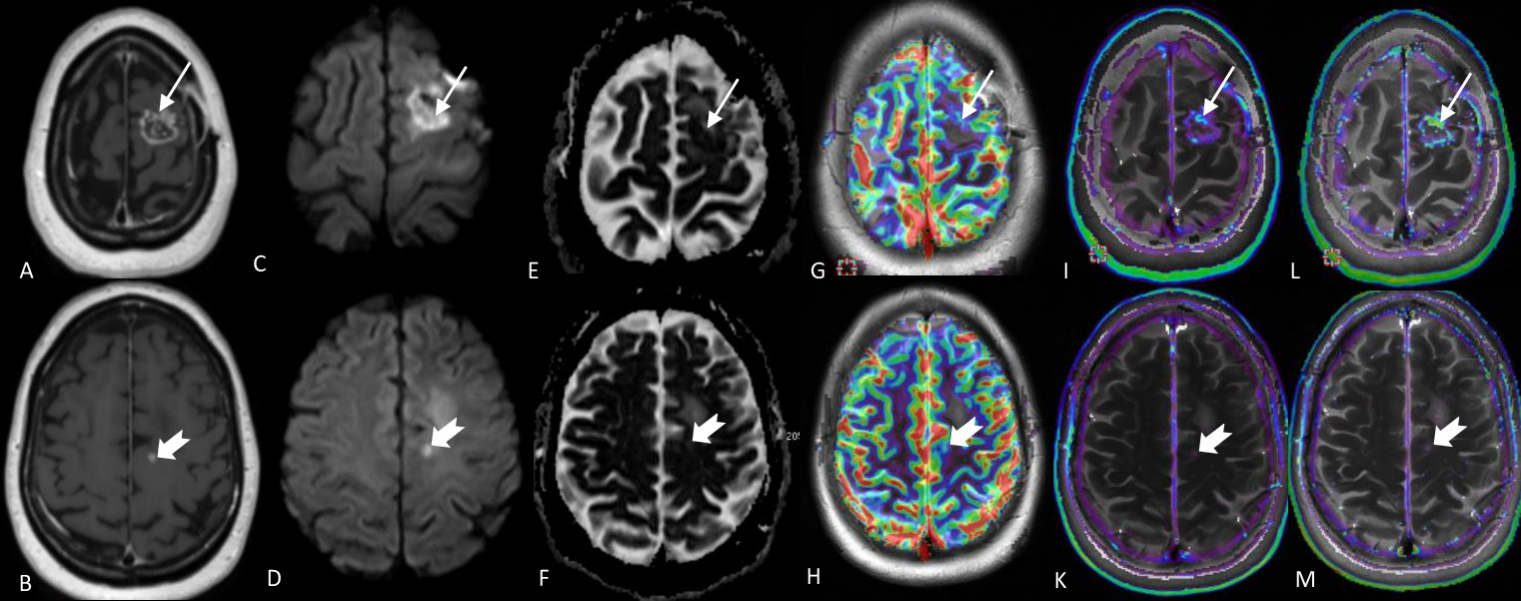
Multiparametric MRI in a patient with GBM on the left frontal lobe treated with surgery and adjuvant radiation with concurrent temozolomide. The arrows on the images in the upper row demonstrate the ring-enhancing treated tumour bed (A) with strong diffusion attenuation (C, E) due to presumable necrotic material and blood and punctate increase in the relative cerebral blood volume (G) and permeability-Ktrans (J) maps. The interstitial volume map (L) shows significant, treatment-related increase in the extravascular extracellular space (ve). The adjacent slice (B) shows punctate enhancement (notched arrow) with strong diffusion attenuation and perifocal oedema (D,F). The enhancing focus shows also increased vascularity in the relative cerebral blood volume (H) and permeability- Ktrans (K) maps, though its conspicuity is less on the DSC imaging (notched arrows). The lesion demonstrates also pathological “ve” values (M). The final diagnosis suggests predominantly treatment-related changes with foci of tumour residual/recurrent disease. DSC, dynamic susceptibility contrast.
